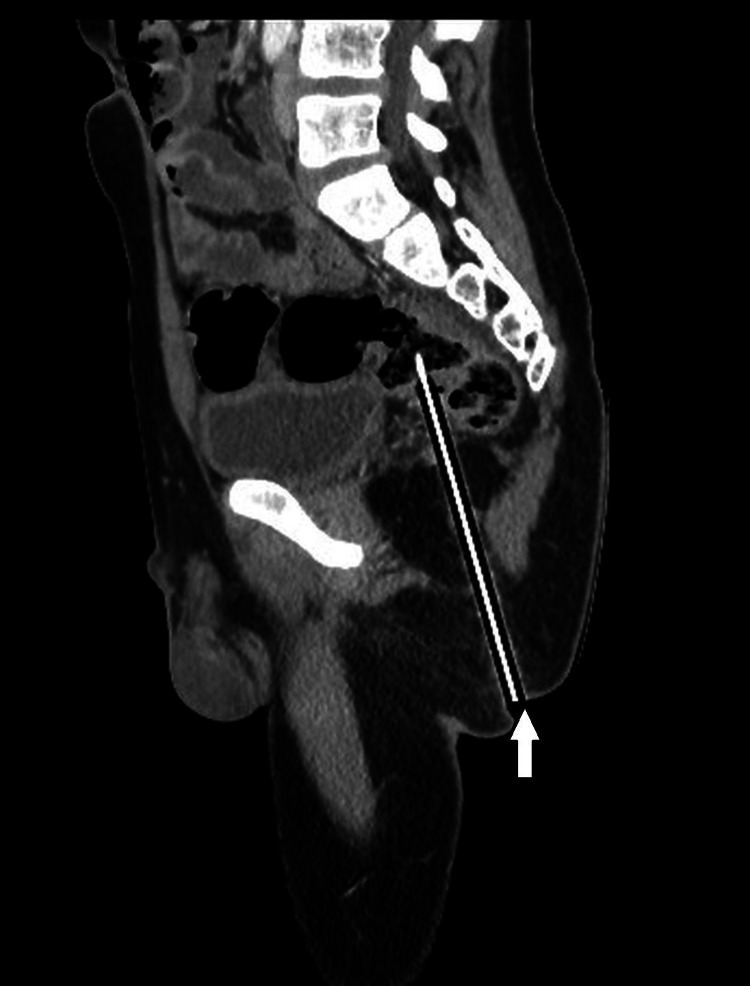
Sagittal CT reconstruction shows the full trajectory of the pencil (white arrow) with the tip appearing to project toward or into the rectosigmoid lumen, indicating a possible intraluminal position.
